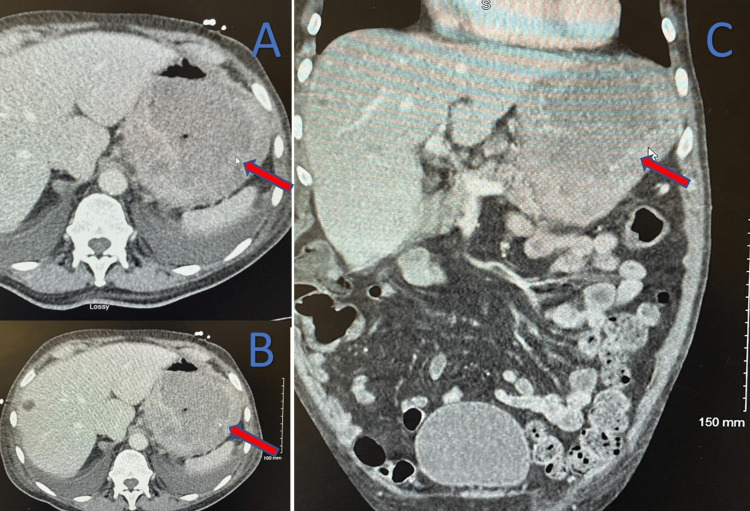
CT showing thickened gastric mucosa (red arrows). A and B axial images. C coronal image.
